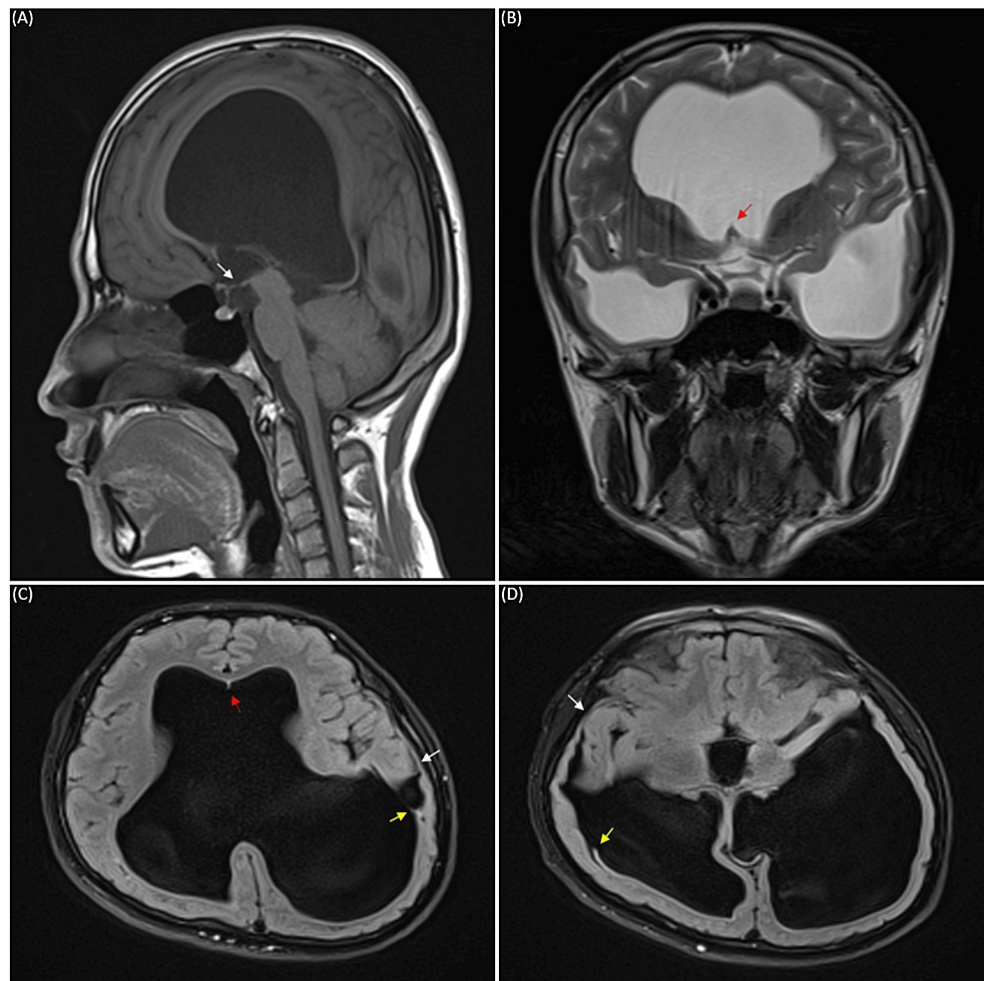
MRI images of the patient’s head. (A) T1-weighted sagittal view. (B) T2-weighted coronal view. (C) and (D) Fluid-attenuated inversion recovery (FLAIR) axial view. White arrows indicate spontaneous ventriculostomy, yellow arrows indicate the rupture of ventricular walls, and red arrows indicate remnants of the septum pellucidum.
